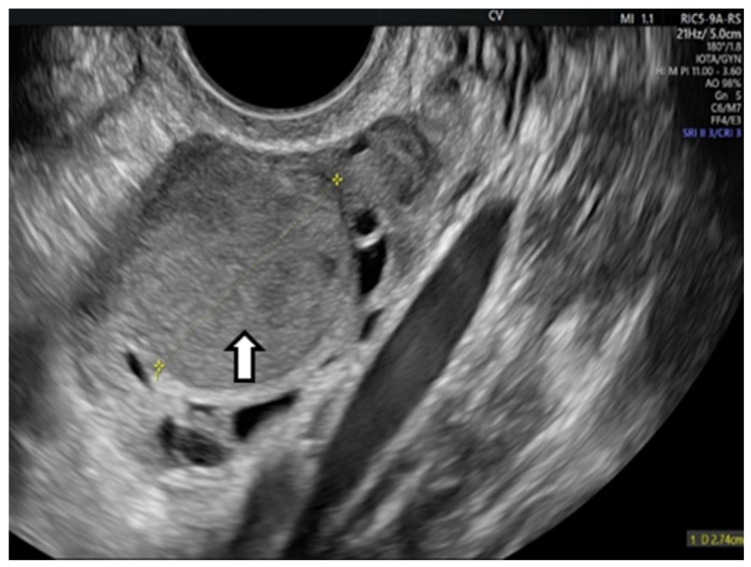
Vaginal ultrasound image of the ovary. The arrows show the ovarian endometrioma with ground-glass appearance.
